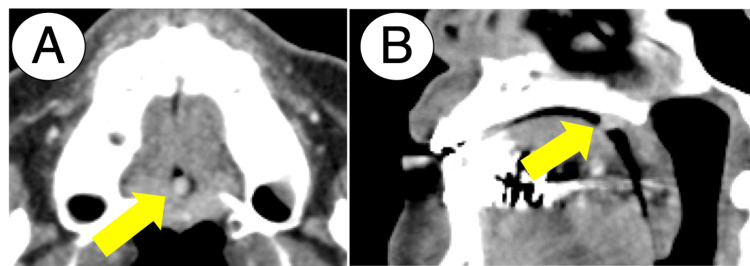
Contrast-enhanced computed tomography: (A) axial view and (B) coronal view
